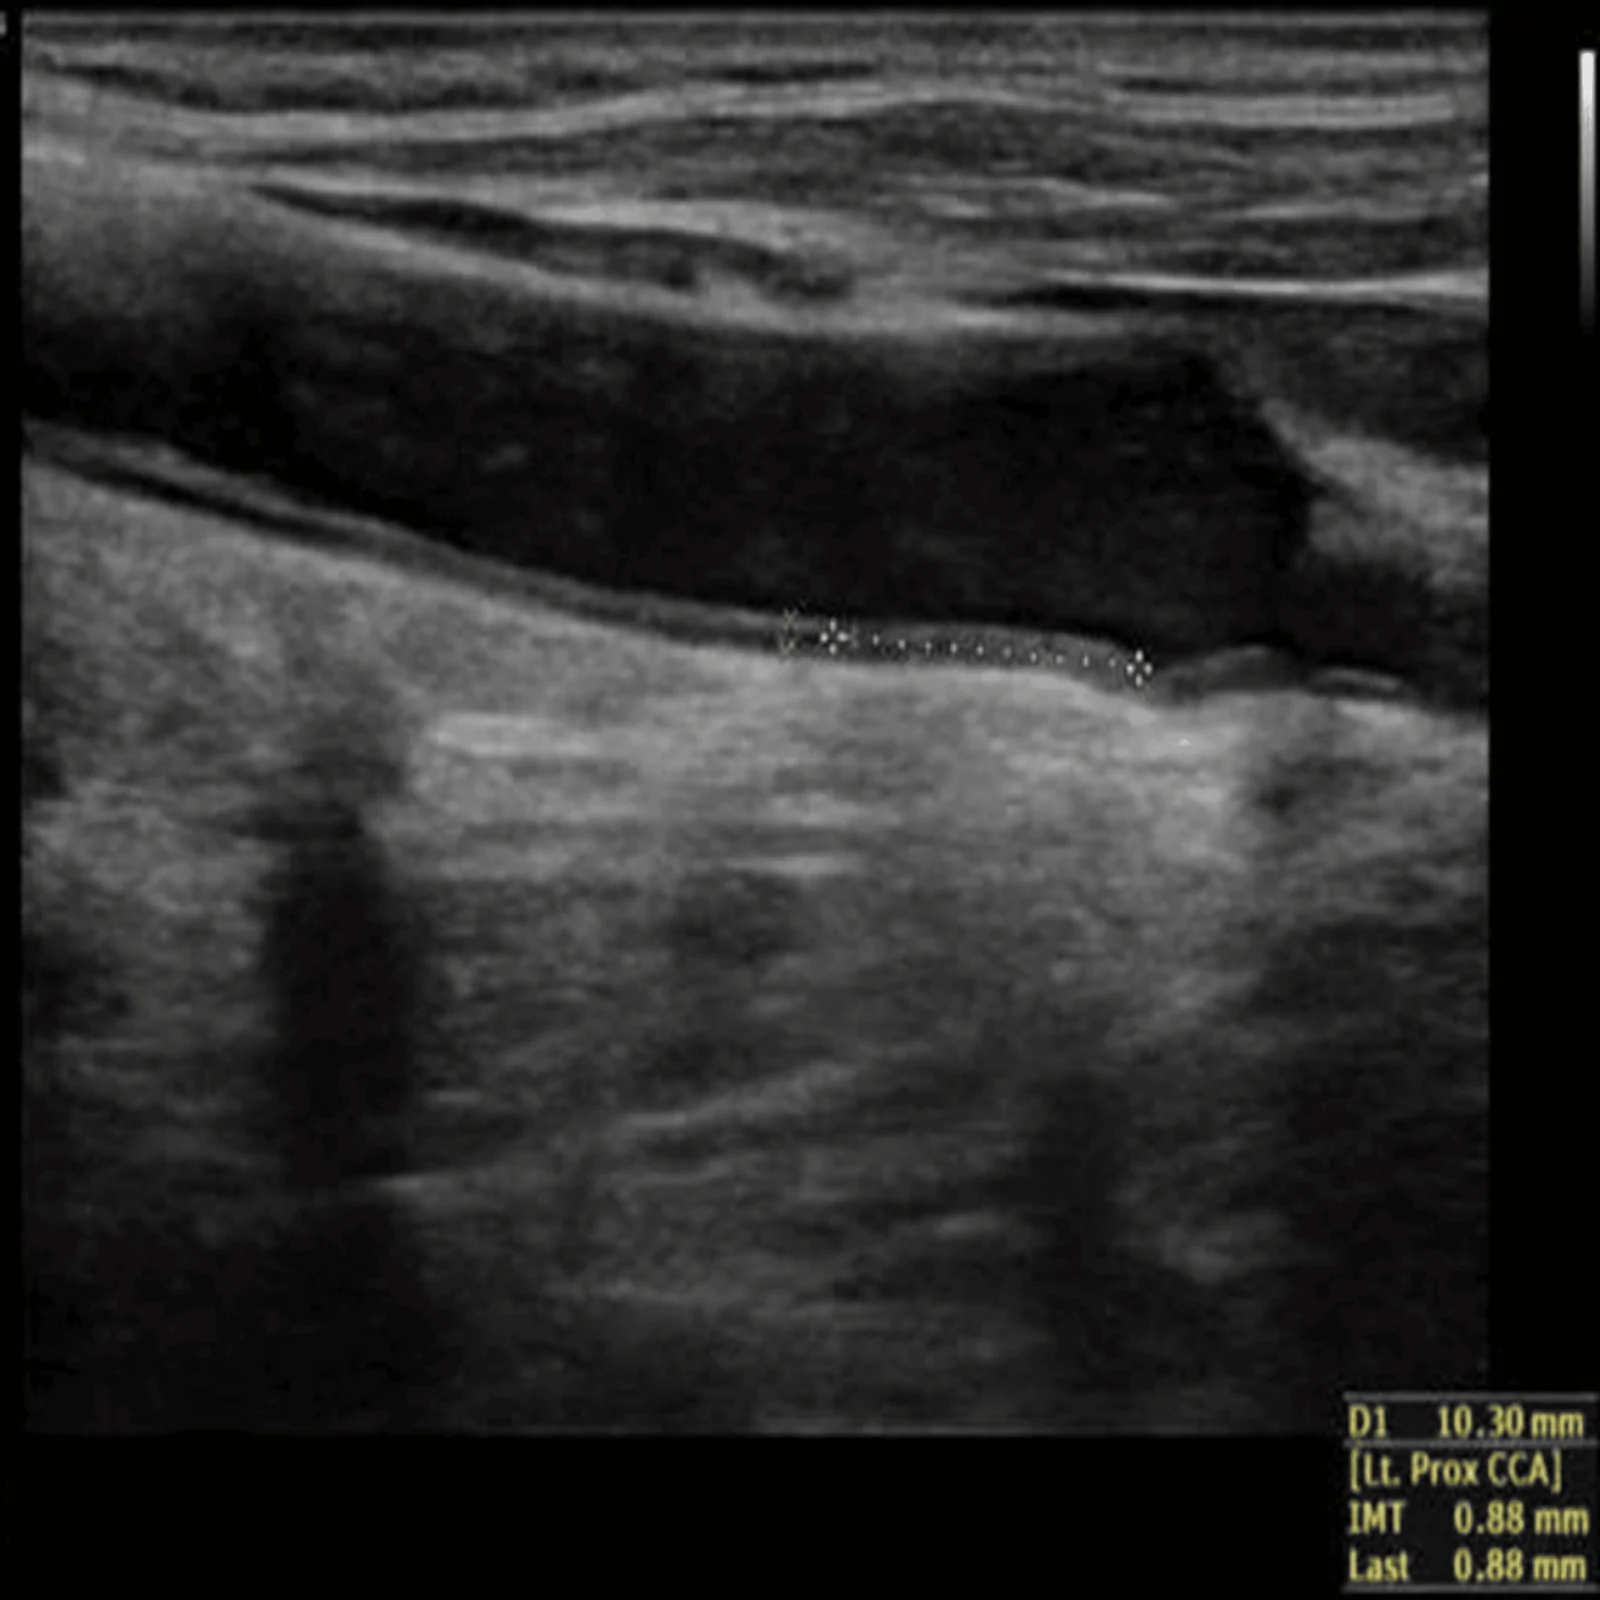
B-mode ultrasound of the left proximal common carotid artery demonstrating far-wall CIMT measurement of 0.88 mm. CIMT: Carotid Intima-Media Thickness.
